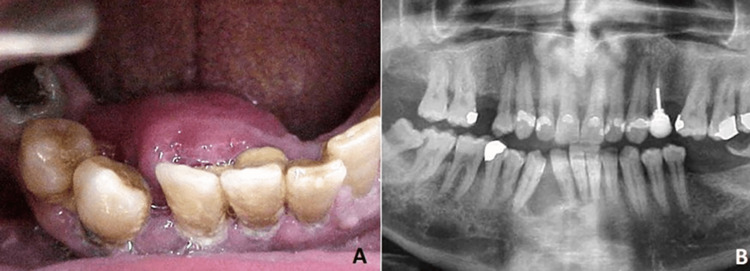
A: Intraoral photograph of the patient showing the expansion of the lingual cortical plate at the anterior mandible. B: Original orthopantomogram of the patient.
